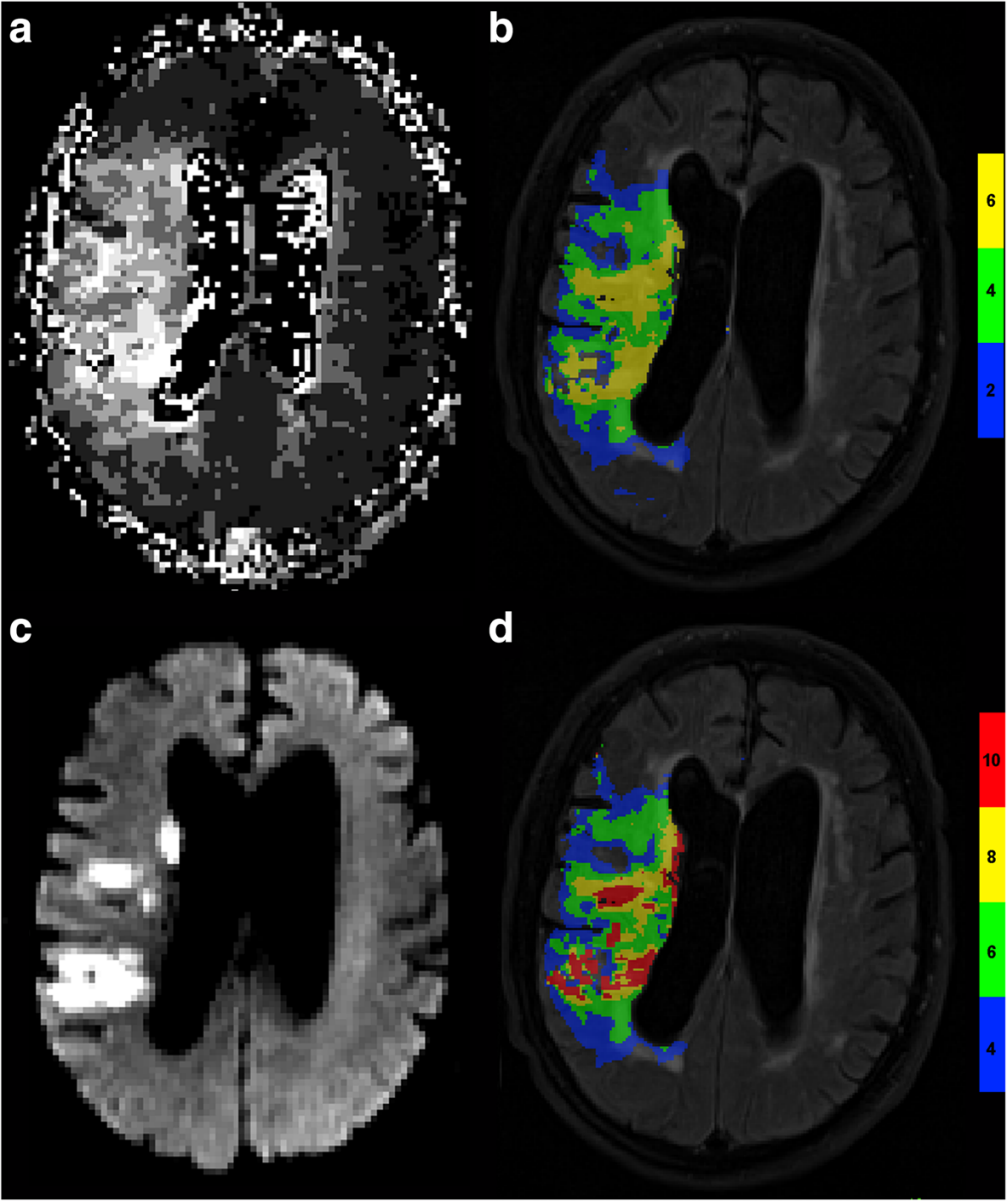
Patient with 90 % carotid artery stenosis and unfavorable outcome. a Large prolonged TTP volume on TTP-map provided by scanner software without thresholding. b Large TTP volume with markedly prolonged TTP on TTP-map calculated by Olea-Sphere®. c DWI provided by scanner software showing multiple areas with restricted diffusion. High grade intracranial stenosis or occlusion was excluded in angiography. d Large prolonged T_max_ volume calculated by Olea-Sphere®
